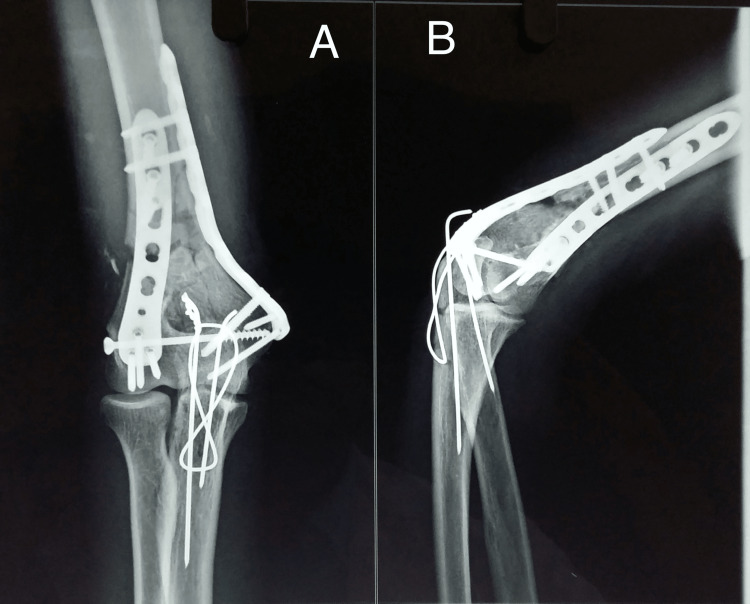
Three-month postoperative follow-up radiograph. Third-month postoperative follow-up radiograph showing the progressive union of the fracture and olecranon osteotomy. A: anteroposterior view; B: lateral view.
